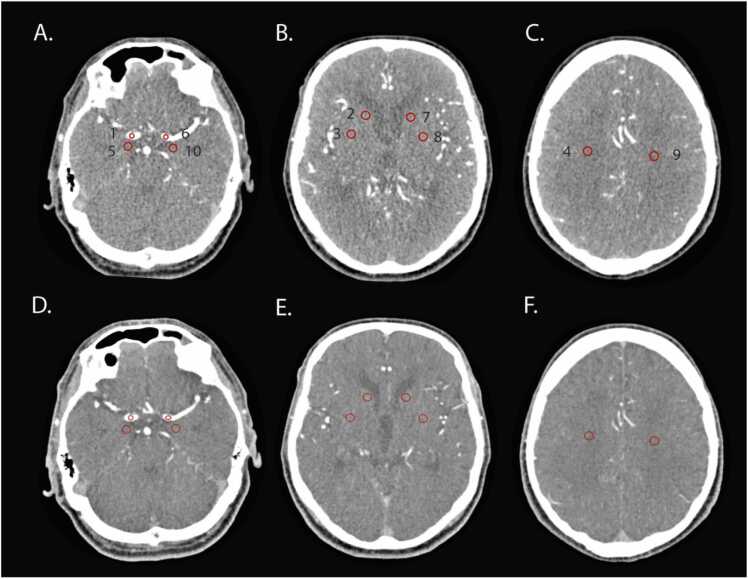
Location of both ipsi- and contralateral measurements on CTP-AR and CTA. Images A-C correspond to the CTP-AR, images D-F correspond to the CTA. The ROI were placed on the following ipsilateral sites: (1) middle cerebral artery (M1) of 10 mm^2^, (2) caudate nucleus of 30 mm^2^, (3) lentiform nucleus of 30 mm^2^, (4) centrum semiovale of 30 mm^2^, (5) the parenchyma adjacent M1 of 30 mm^2^. ROI 6–10 were placed on the same locations but on the contralateral side. Abbreviations: CTA=computed tomography angiography; CTP-AR=computed tomography angiographic reconstructions; ROI=region of interest.
